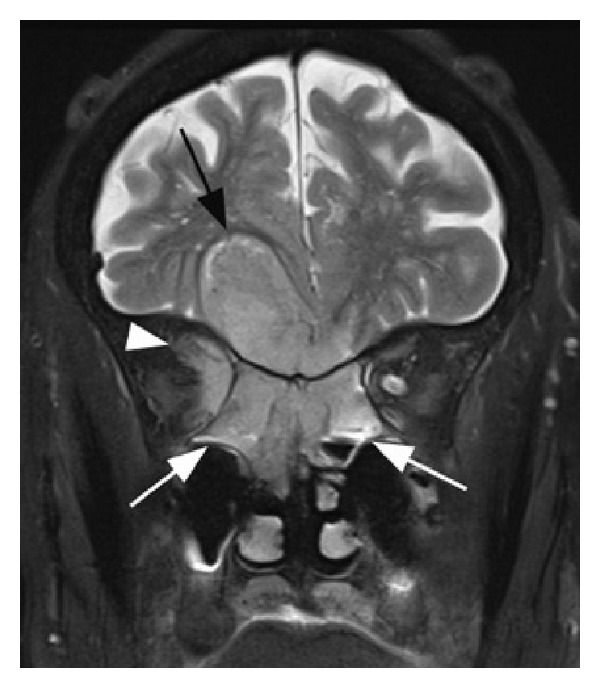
Coronal T2-weighted brain MRI showing a sinonasal mass involving the ethmoid/sphenoid sinuses (white arrows) with extension into the right orbit (white arrow head) and anterior cranial fossa (black arrow).
